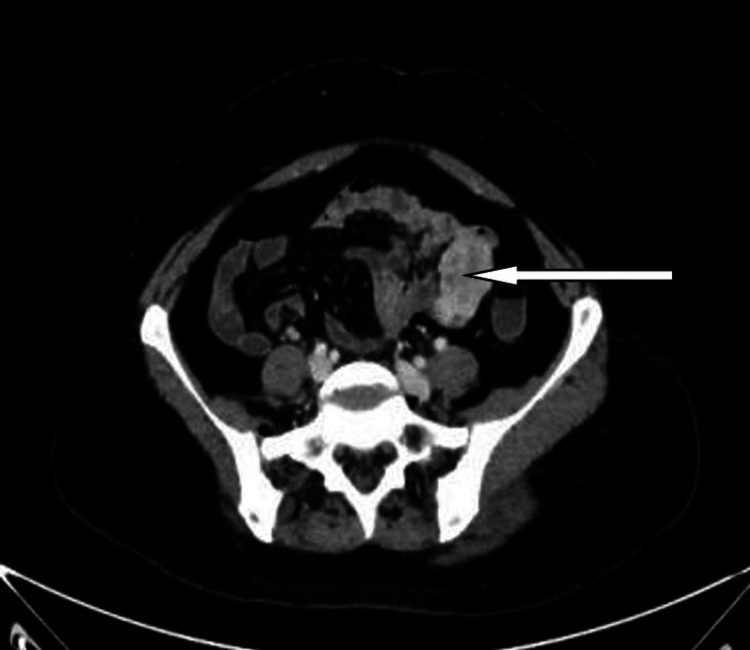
CT scan angiogram image showing a 4 x 3.7 x 3 cm heterogeneously enhancing soft tissue lesion arising from the proximal jejunum (the arrow).
